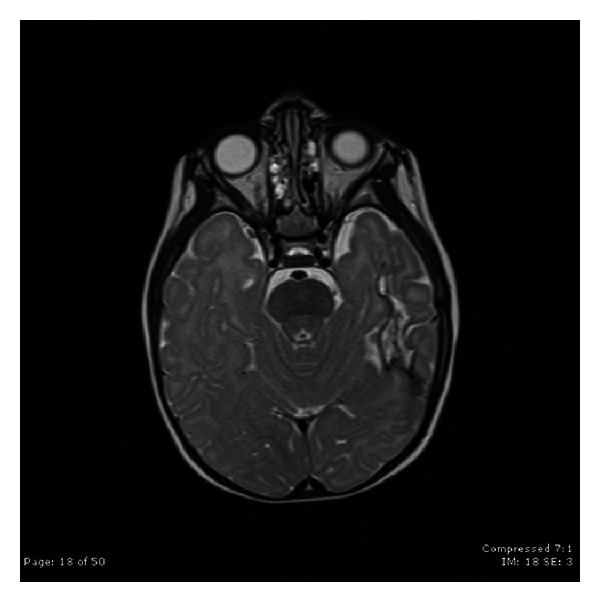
Axial T2-WI MRI scan demonstrating congenital vascular malformation in the left temporal lobe.
